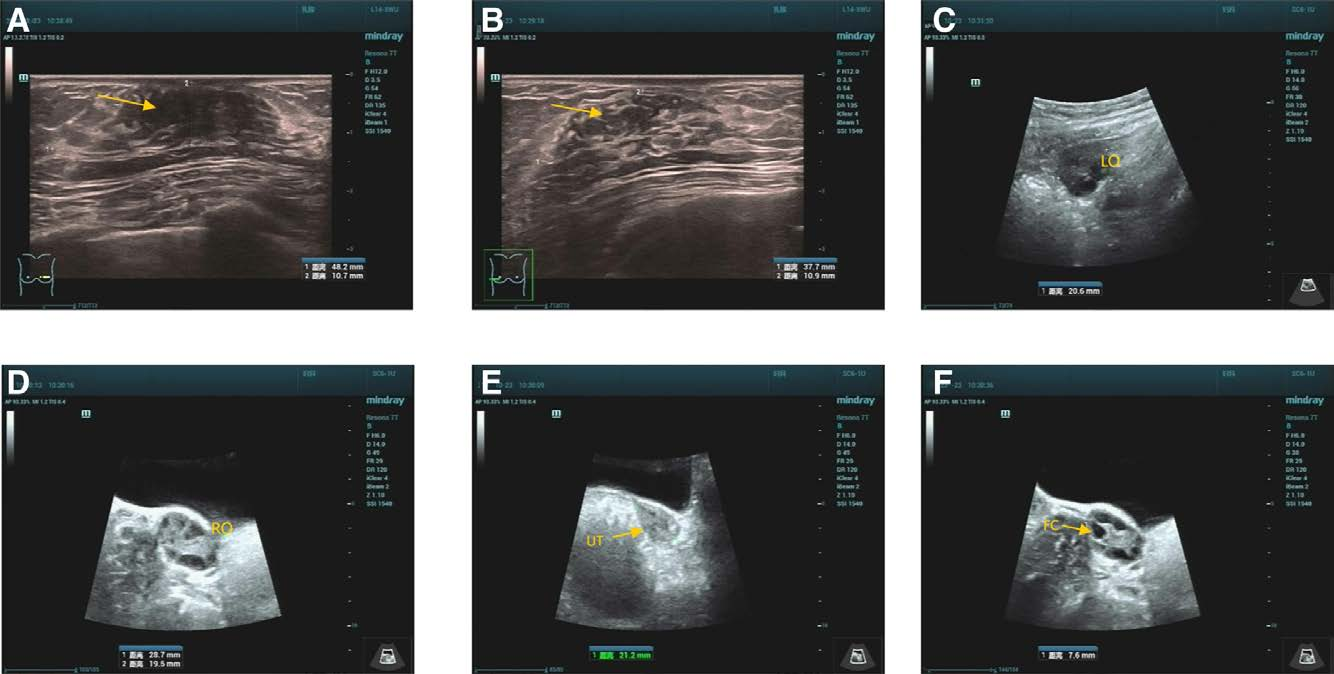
Ultrasound scans on the breasts and reproductive organs. (A) Left breast (size: 48.2 mm × 10.7 mm); (B) right breast (size: 37.7 mm × 10.9 mm), yellow arrows in (A, B) indicate the mammary glands; (C) left ovary (LO, size: 30.8 mm × 17.3 mm × 20.6 mm); (D) right ovary (RO, size: 28.7 mm × 19.5 mm × 25.9 mm); (E) uterus (UT, length: 46 mm); (F) follicles (FC). FC = follicles, LO = left ovary, RO = right ovary, UT = uterus.
